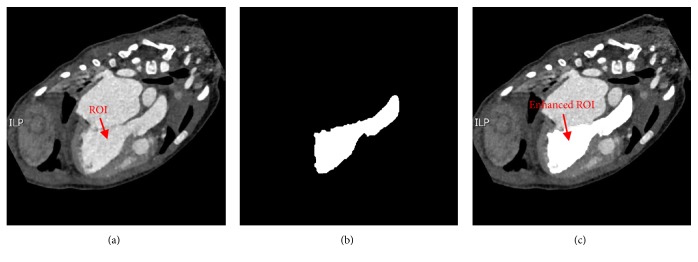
The process of region enhancement. (a) Original CT image *I*_*R*_(*x*). (b) Enhancement matrix *V*_*R*_(*x*). (c) Enhanced CT image. CT, computed tomography; ROI, region of interest.
